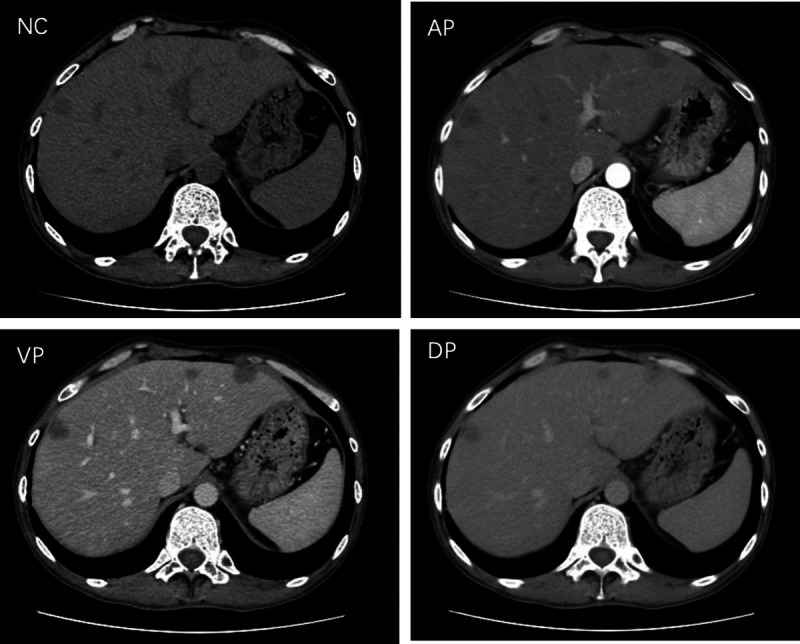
Abdominal contrast-enhanced CT suggested multiple hypodense lesions in the liver, with mild enhancement on the contrast phase. Small blood vessels appeared to pass through the lesions during the arterial phase. NC: Noncontrast; AP: Arterial phase; VP: Venous phase; DP: Delayed phase.
